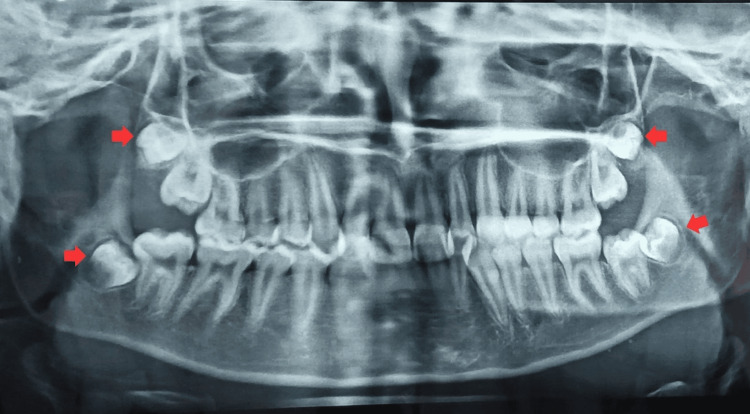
An orthopantomogram showing developing third molars in both maxilla and mandible
